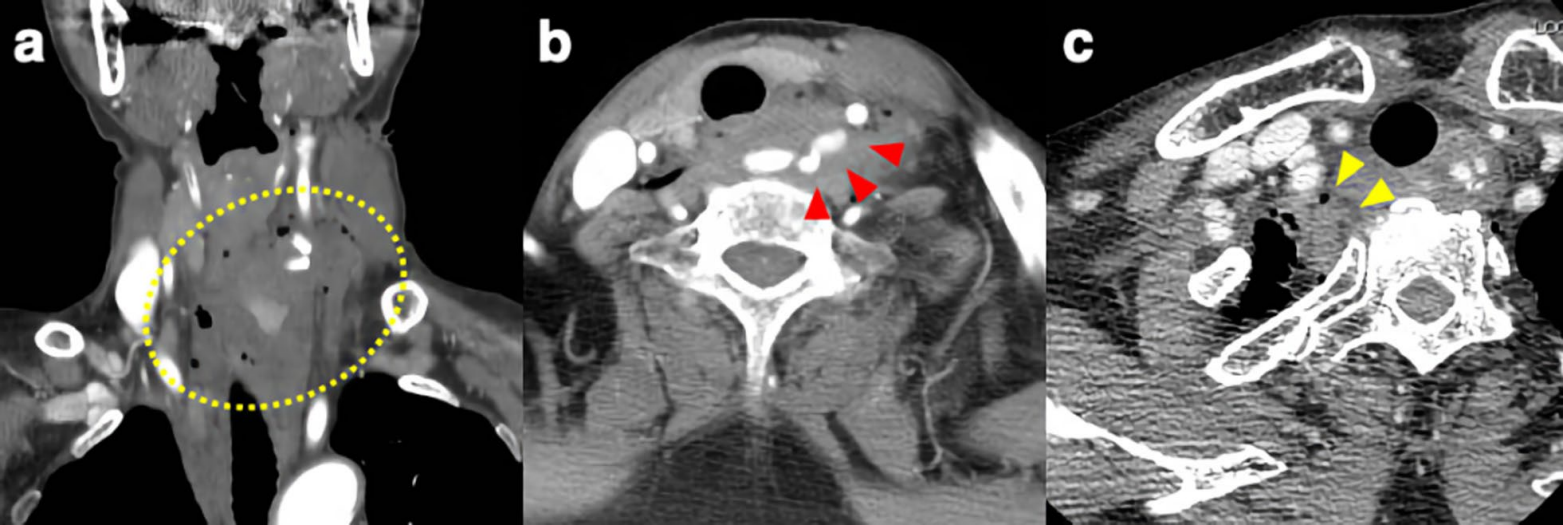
CT at the time of transport to the hospital. a The hematoma was spread from the left neck to the right upper mediastinum (yellow dotted line). b The internal jugular vein was damaged, and extravascular leakage from the vein was observed (red arrowheads). c There was pulmonary contusion in the right upper lobe but no hemopneumothorax (yellow arrowheads)
